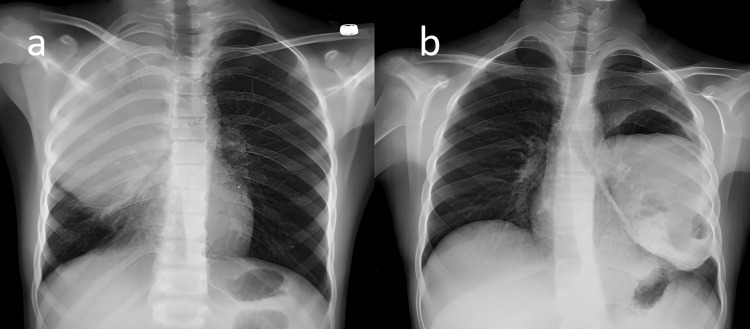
Chest X-ray image of ruptured and non-ruptured pulmonary hydatid cysts (a) Chest X‑ray showing a giant spherical cystic mass (hydatid cyst) displayed in the upper part of the right lung. (b) Chest X-ray showing a cystic mass showing air-fluid level in the lower lobe of the left lung (ruptured hydatid cyst).
